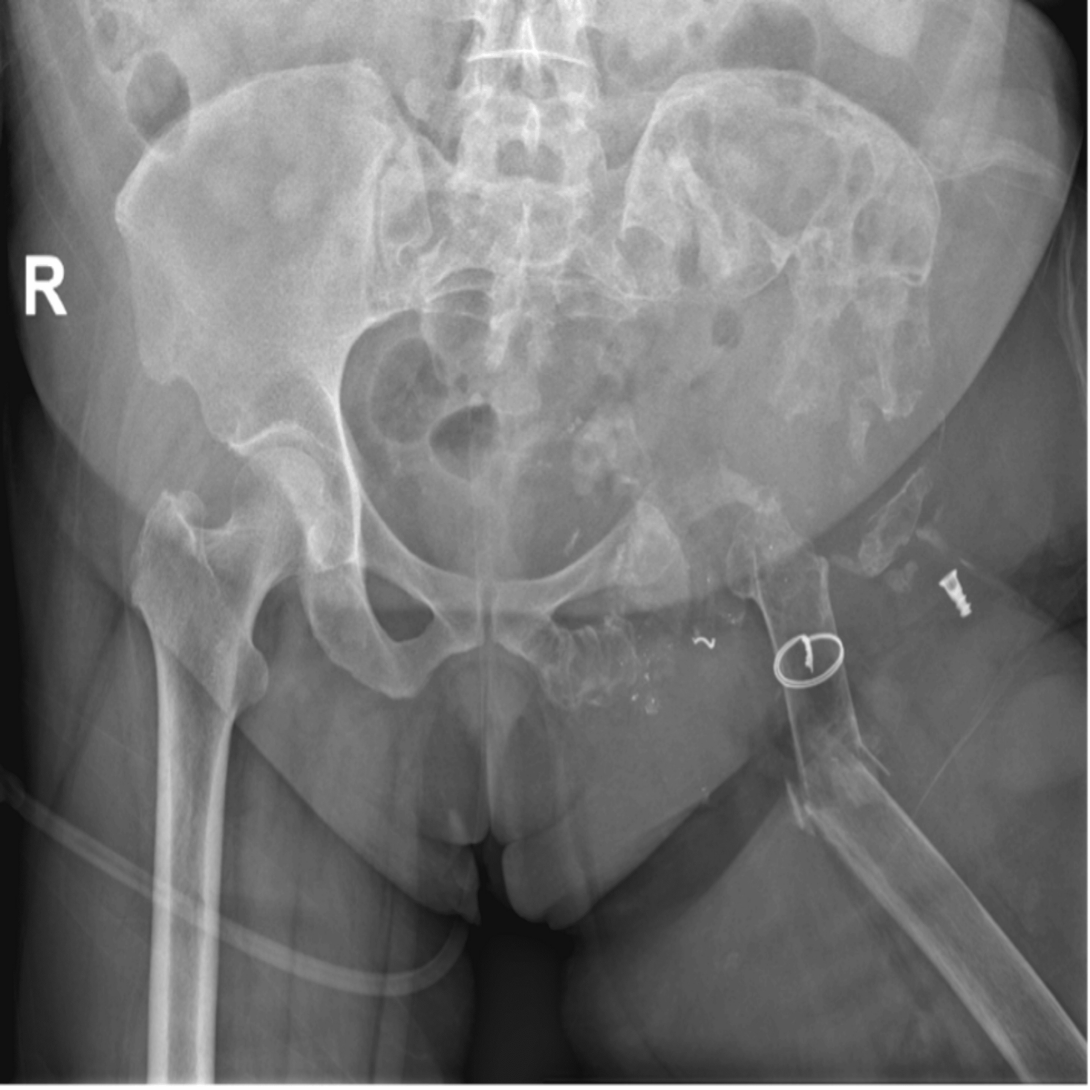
Pre-operative radiograph view of left femur shaft fracture
